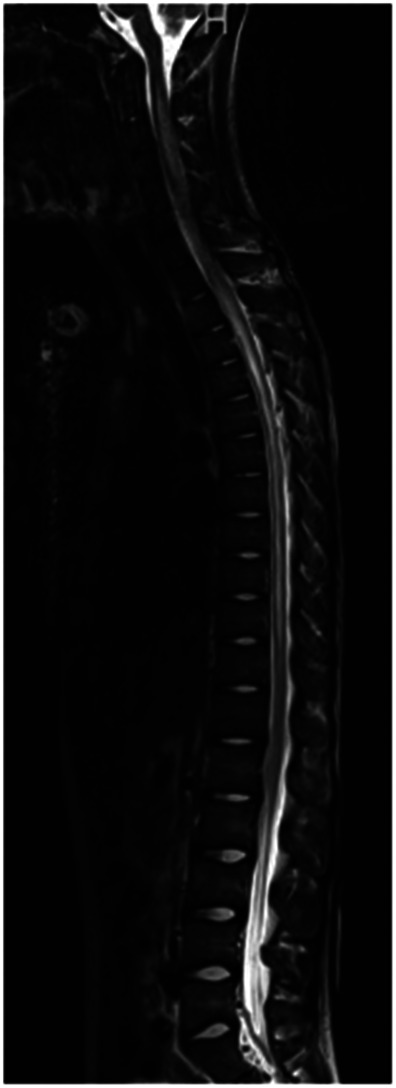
Imaging at the time of presentation to the emergency department. MRI demonstrated extensive T2 signal abnormality involving nearly the entire spinal cord, with hazy enhancement from C5 to T5, as can be seen with transverse myelitis.
